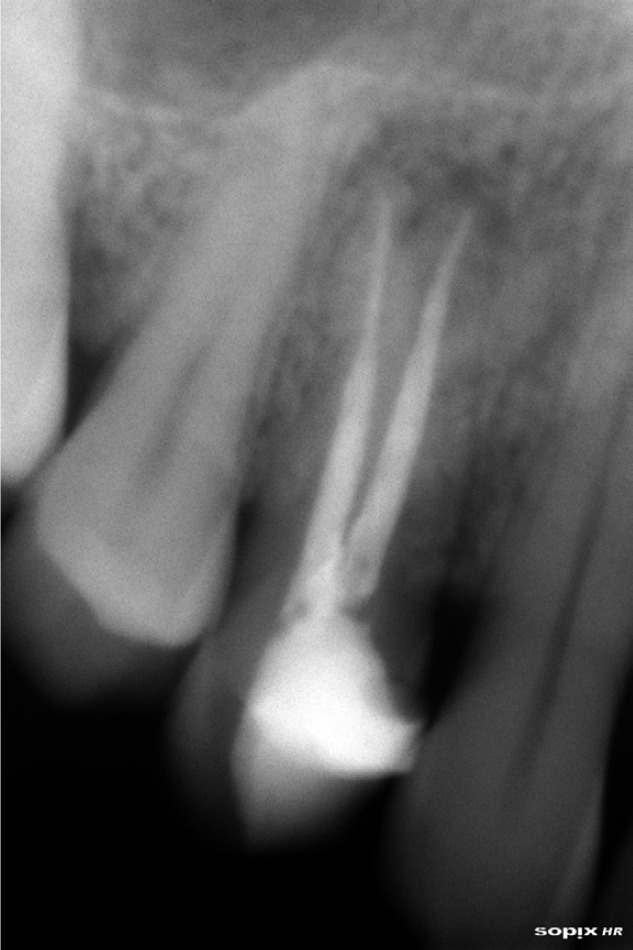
Periapical radiograph show a root canal treatment and periapical lesion.
